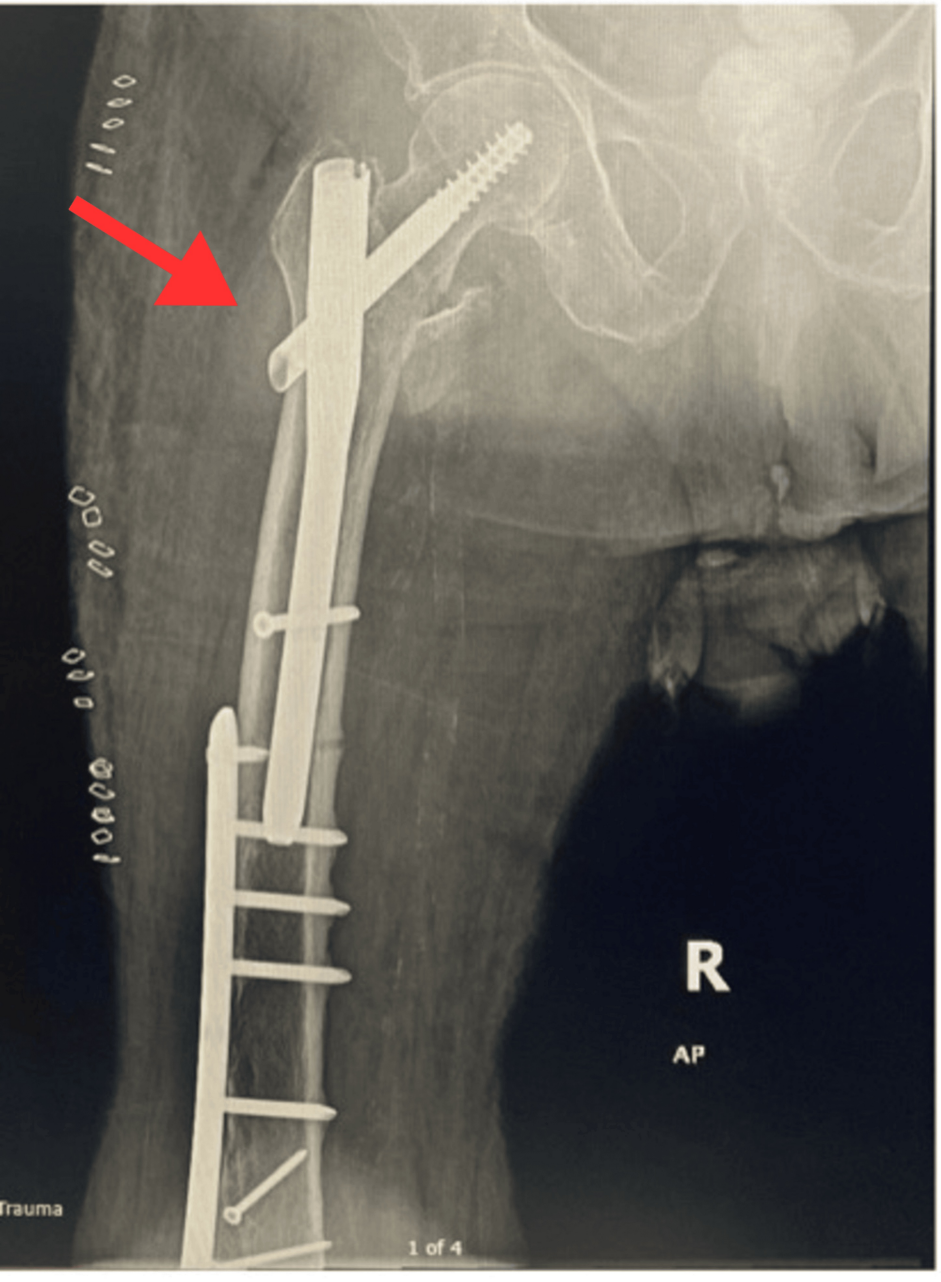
Postoperative right hip X-ray (AP view), 3 June 2024, showing TFNA fixation in place.
